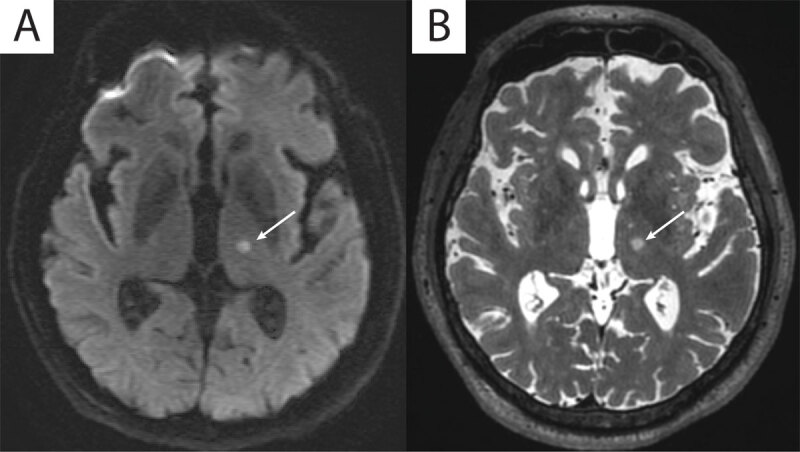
An 80 year-old patient with a typical immediate post-procedural imaging result depicting a spheroid-shaped lesion in the left ventral thalamus with restricted water diffusion. A. diffusion weighted imaging and B. hyperintense signal on axial T2-weighted imaging.
